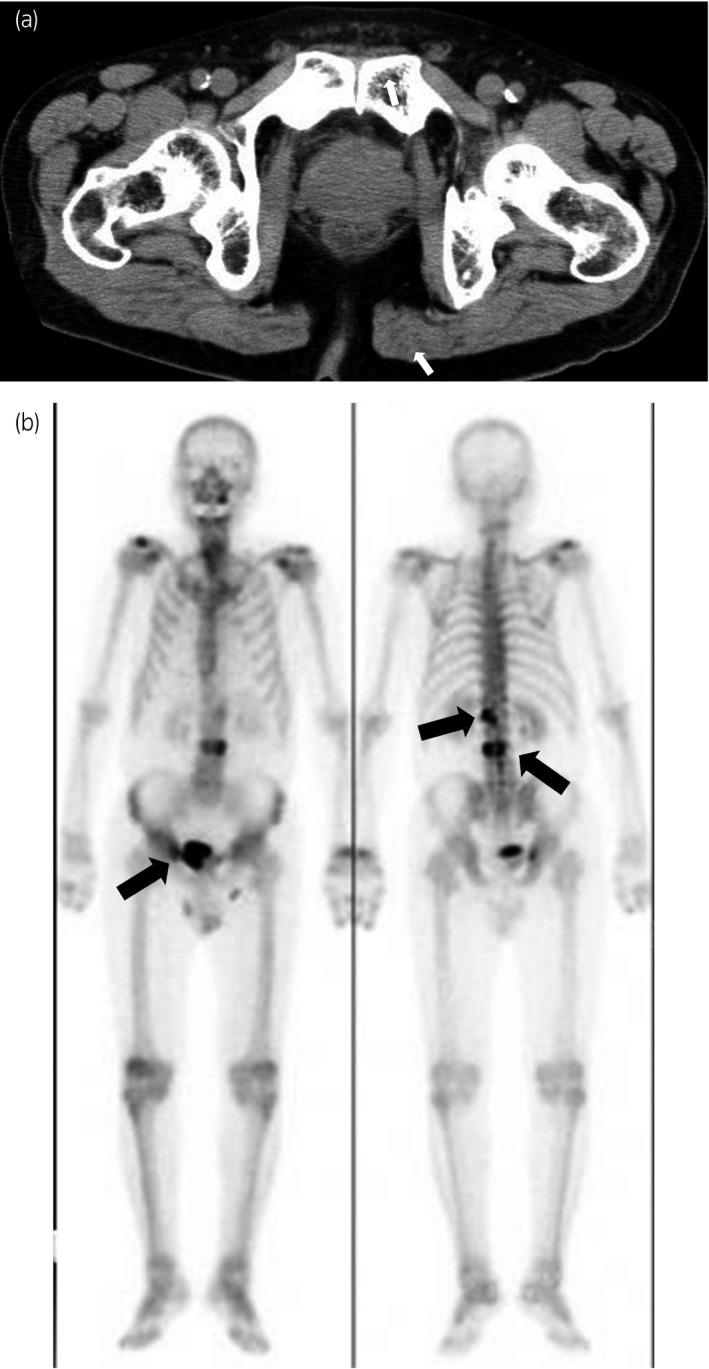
(a) Computed tomography image showing an irregular enlarged prostate in the left lobe (arrows). (b) Bone scintigraphy image showing multiple bone metastases (arrows).
